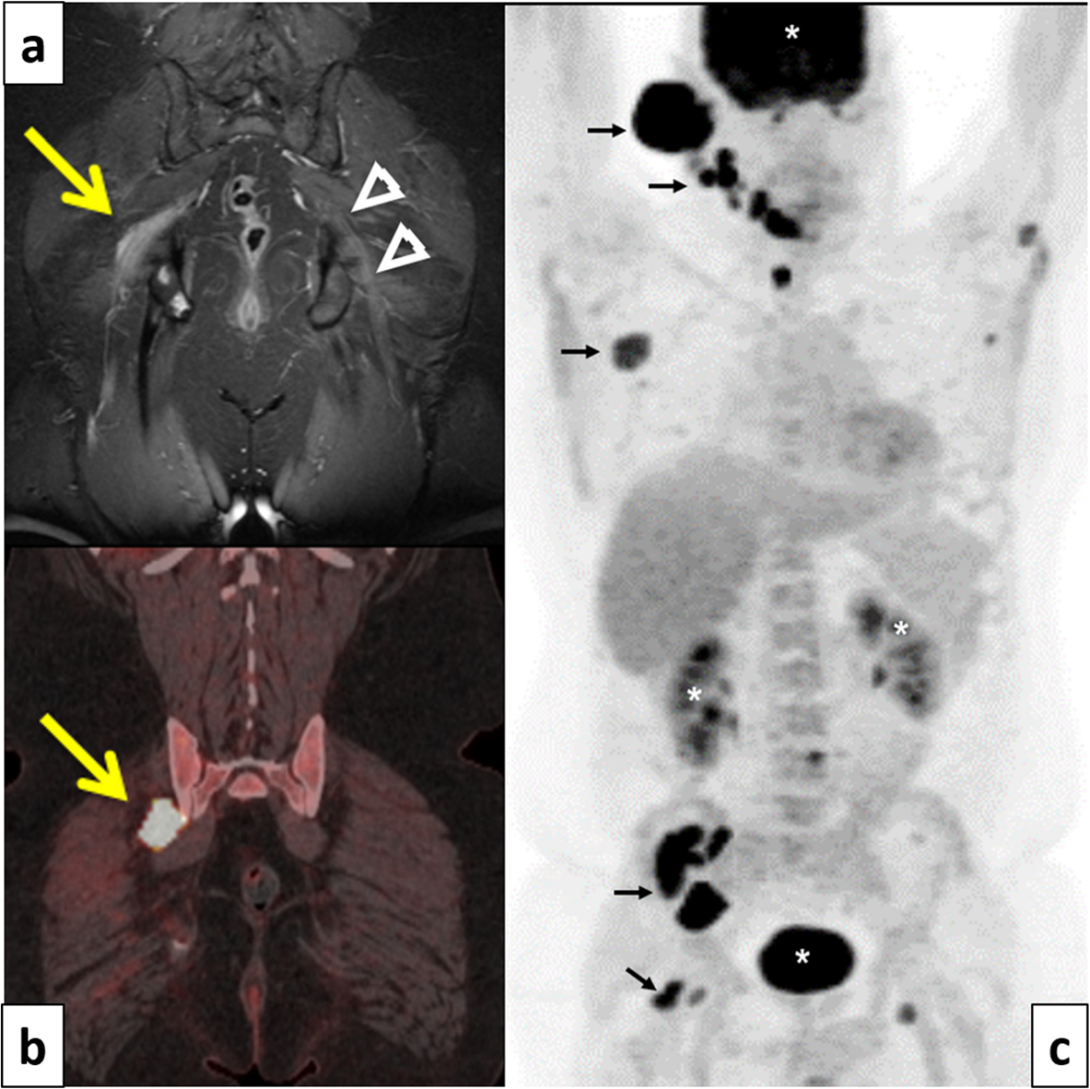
Case 1. 44 years old man. a Pelvis coronal contrast-enhanced, fat-suppressed T1- weighted image. Thickening and enhancement of the proximal right sciatic nerve (arrow). Normal contra-lateral side sciatic nerve for comparison (arrow heads). b Coronal fused F-18 FDG PET/CT image demonstrating an area of intense hypermetabolic focal activity in the region of the right lumbosacral plexus, in keeping with nodular neurolymphomatosis. c Maximum intensity projection (MIP) F-18 FDG PET/CT image demonstrating widespread nodal and extra-nodal hypermetabolic foci (arrows). Note that the intense hypermetabolic activity within the brain, kidneys and urinary bladder are normal findings (*)
